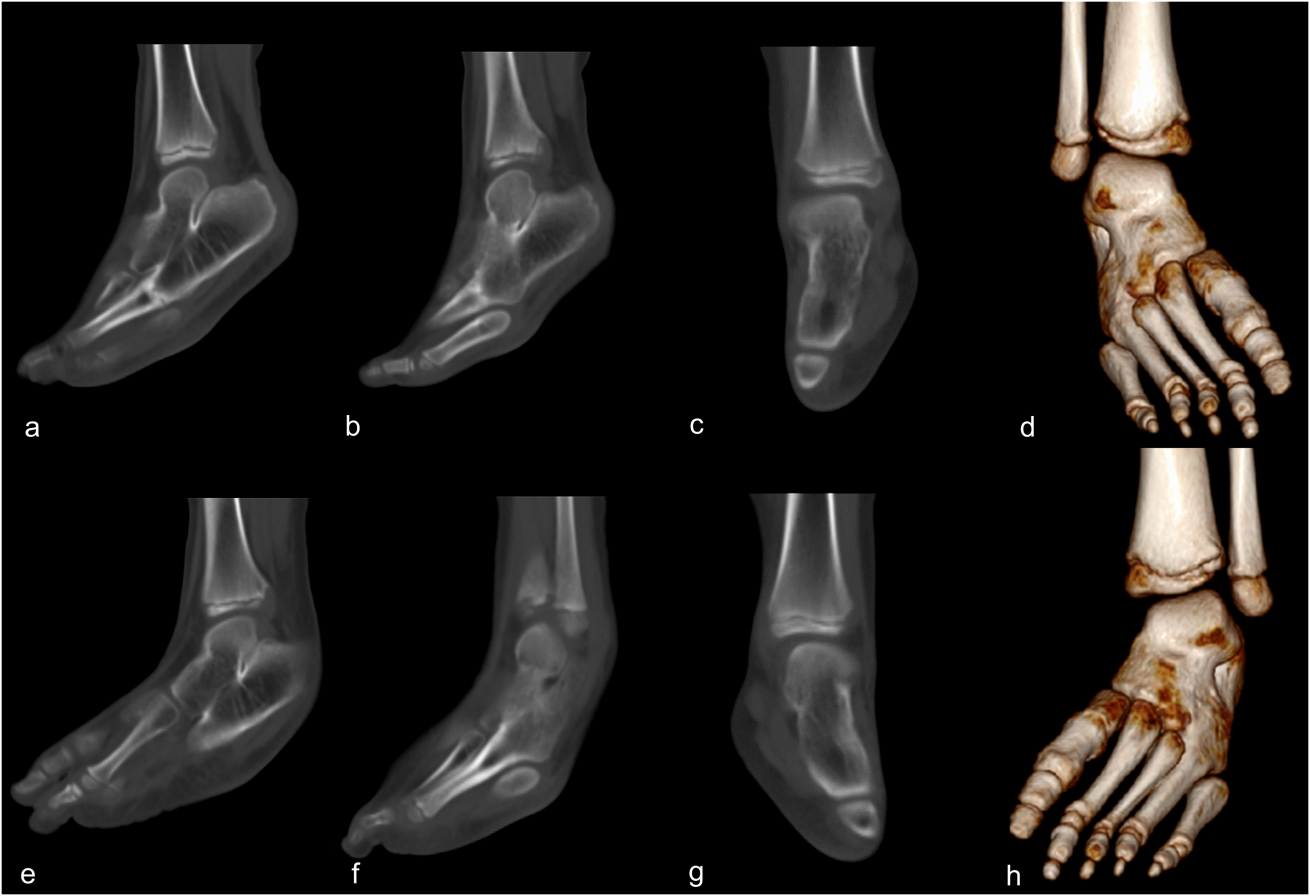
Computed tomography scan of a 5-year-old boy with bilateral massive tarsal coalitions. The sagittal views of the right foot show the osseous unions of the tarsal bones (a) and the union extending to the fourth metatarsal bone (b) The coronal (c) and 3D reconstructed (d) images of the right foot. The sagittal views of the left foot show the osseous unions of the tarsal bones (e) and the union extending to the fourth metatarsal bone (f). The coronal (g) and 3D reconstructed (h) images of the left foot.
